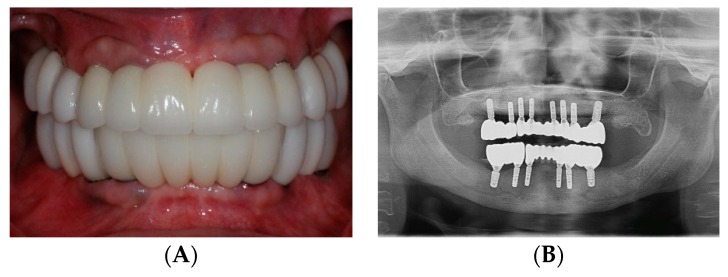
Definitive prosthesis. (A) Intraoral view. (B) Panoramic radiograph.
